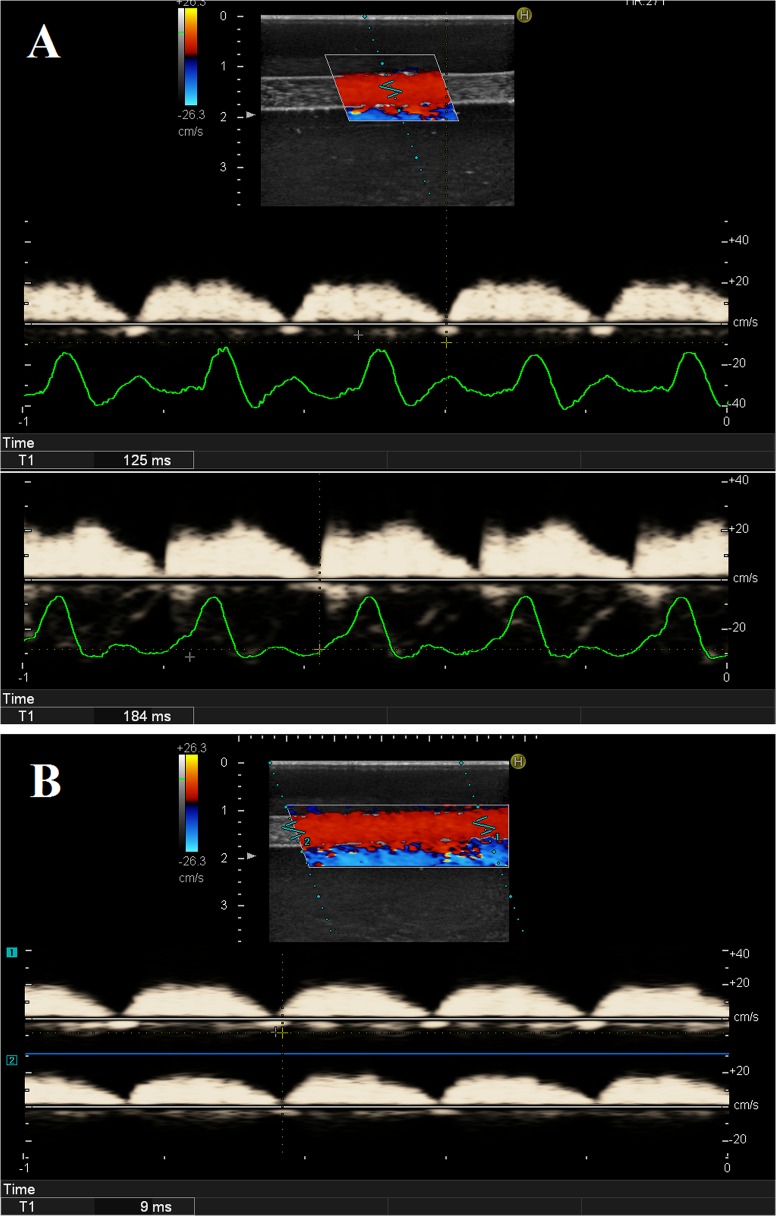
The methodology of the in vitro study. Panel A, the PWV_A-B_ measurement, the top panel was the two dimensional echocardiographic imaging. The velocity spectra recorded at point A and B by pulse wave Doppler were displayed at the middle and bottom, respectively. The pressure curve recorded by the pressure transducer was shown under each spectrum. The time delay between the transducer and point A and B were respectively measured by foot detection using the intersecting tangent method (125ms and 184ms). Thus the transit time between A and B could be calculated as 184−125 = 59ms. Panel B, the local PWV_M_ measured by the DPWD method, the simultaneously recorded dual velocity spectra were shown. The transit time between these two sites (9ms) was measured by wave foot detection using the intersecting tangent method. PWV_A-B_, pulse wave velocity between point A and B. DPWD, dual pulse wave Doppler; PWV_M_, pulse wave velocity at the middle of point A and B.
